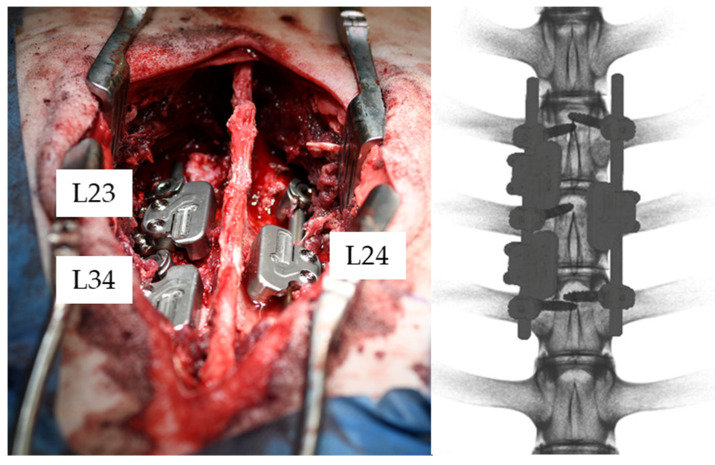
(Left) Intraoperative image after spinal instrumentation and placement of the sensors (L23, L34, L24). (Right) Posterior-anterior view from the postoperative CT scan (L1–L5).
